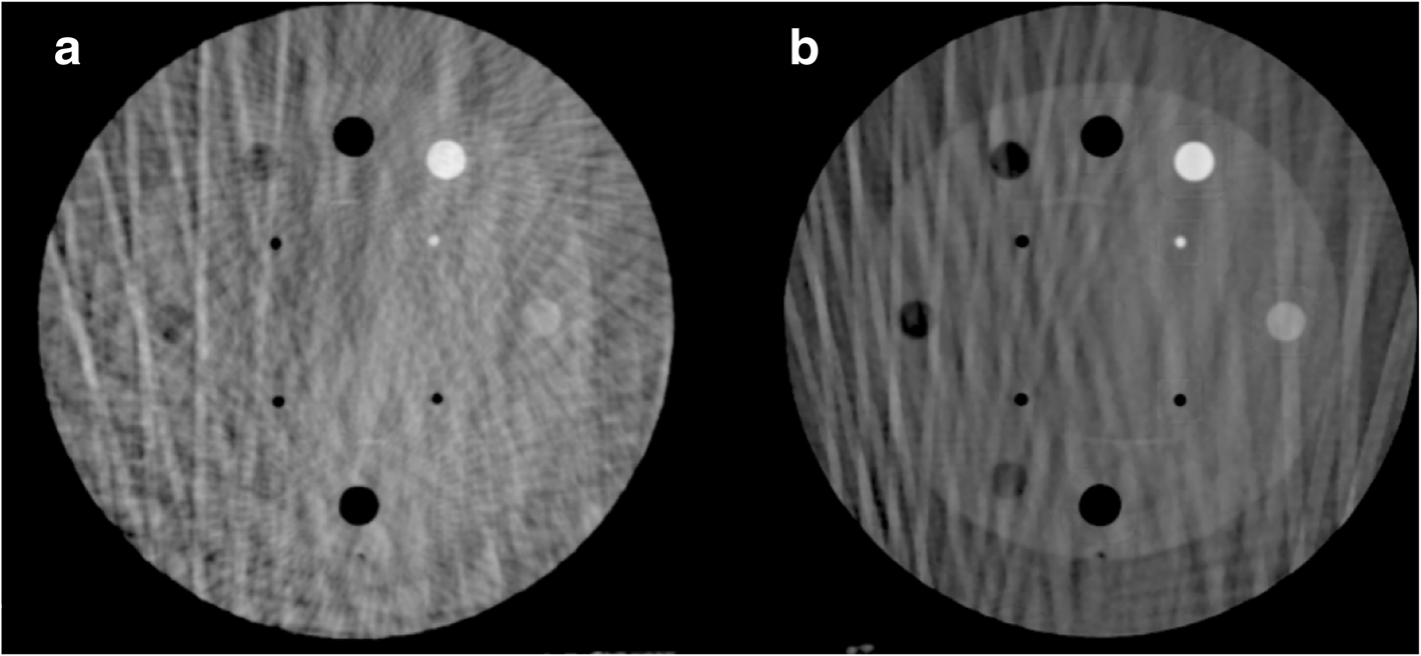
Streaking artifacts present in the sensitometry module for the same CBCT slice and the same phase. These slices correspond to a CBCT-4 ph and b CBCT-10 ph for a gantry speed of 1°/s
